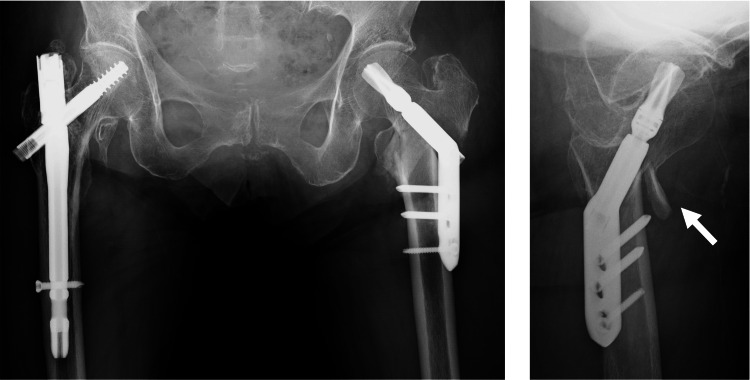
X-ray images of the hip one week postoperatively. Over-telescoping of the lag screw was observed, and the anterior medial bone fragment was observed in the lateral image (white arrow).
